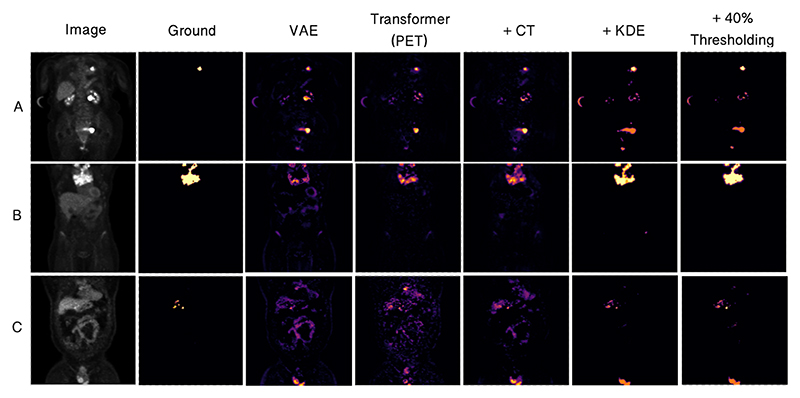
Columns from left to right display (1st) the input image; (2nd) the gold standard truth segmentation; (3rd) the abnormality map as the residual for the VAE, (4th) Transformer, and (5th) CT conditioned methods; (6th) the abnormality map as a KDE, (7th) and after thresholding at 40% of each abnormal region maximum value. Results are provided for four randomly chosen subjects (A,B,C).
